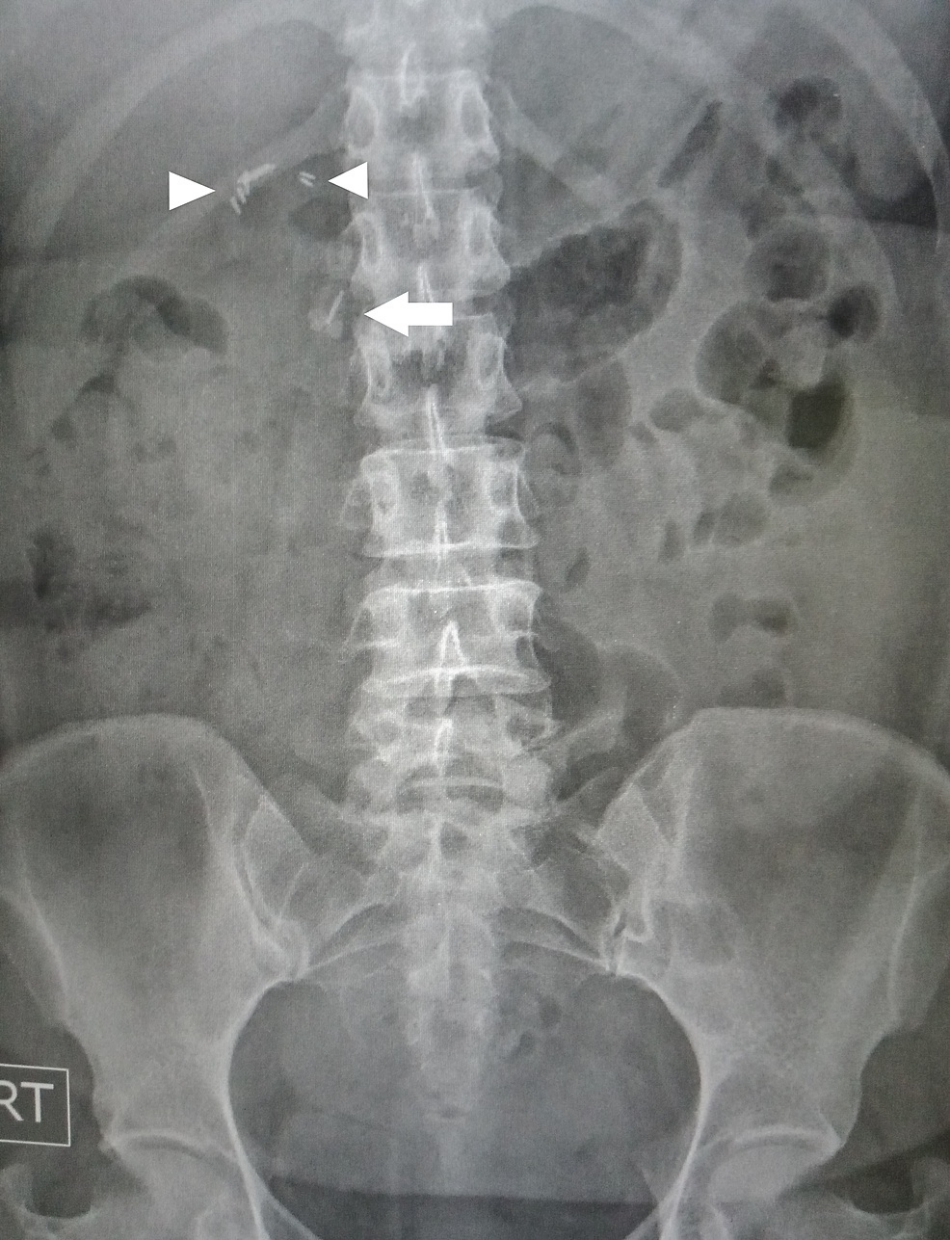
Abdominal X-ray showing surgical clips at level of T12/L1 (arrowheads) and another clip at L1/L2 (arrow). Abdominal X-ray showing surgical clips at level of T12/L1 (arrowheads) and another clip at L1/L2 (arrow).
